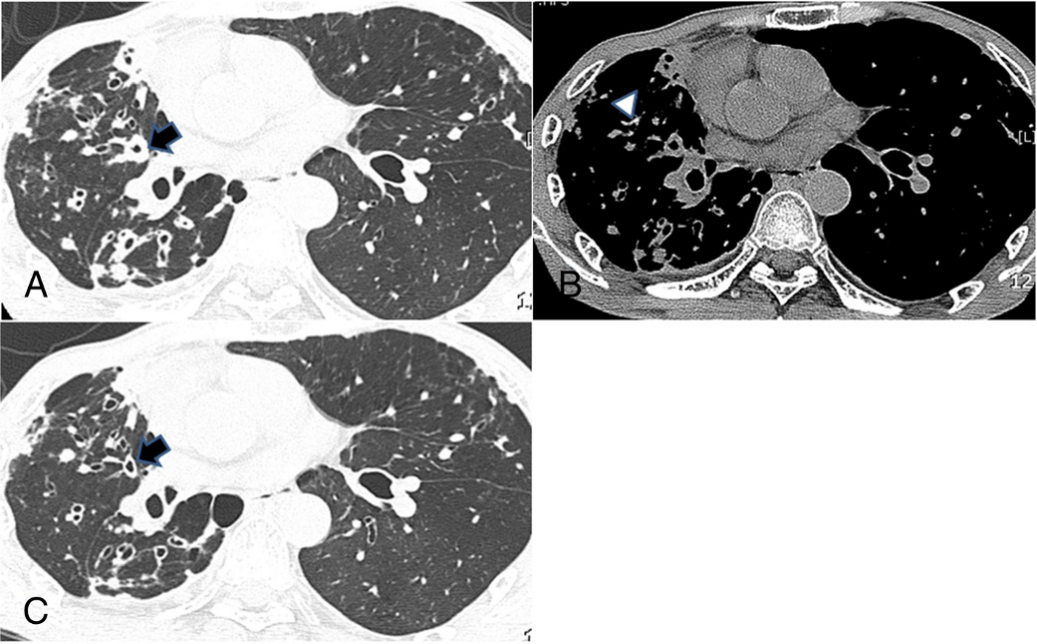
A, B, C Extensively drug-resistant tuberculosis in a 58-year-old man. He had been treated with antituberculosis drugs for 6 months before linezolid treatment. A and B. Transaxial thin-section CT scan shows a cavity (arrow) and bronchial wall thickening in the right upper lobe. Bronchial wall thickening and decreased volume in the right lobe are also noted. Calcified nodule ((B) mediastinal window, arrowhead) and pleural thickening with extrapleural fat proliferation are also observed. C. After 6 months of linezolid treatment, CT scan shows a persistent cavity with a decreased size and thin wall in the right upper lobe (arrow). Bronchial wall thickening improved.
